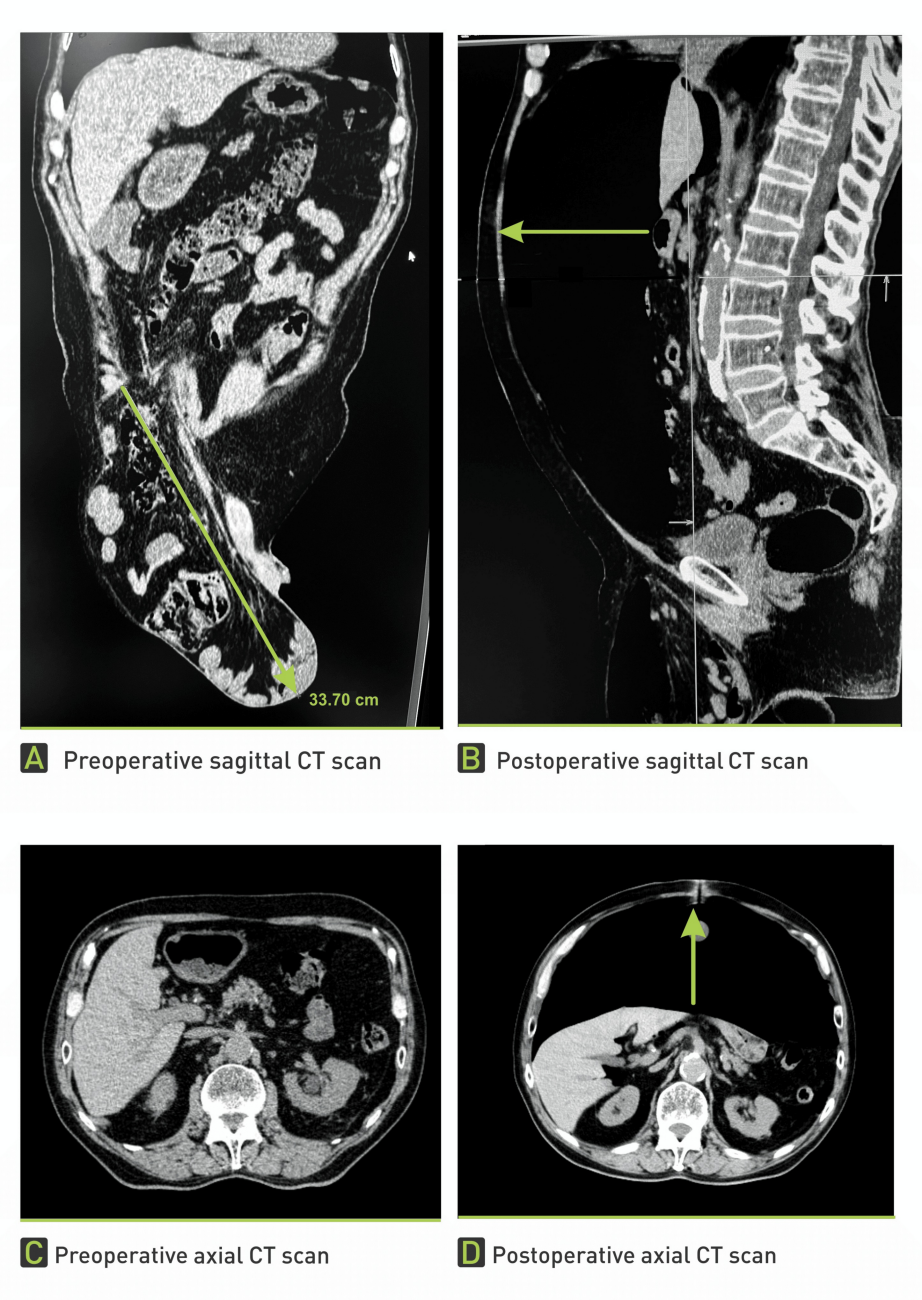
Control CT scan showing a noticeable enlargement of the abdominal cavity (green arrows) and sufficient space created for herniated viscera
